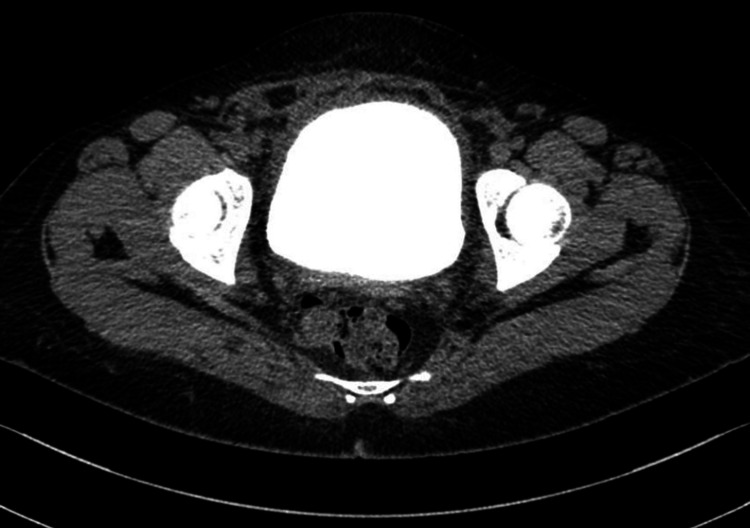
Axial view of the repeated CT cystogram after four weeks from the initial study showing a fully opacified, well-rounded urinary bladder with no evidence of contrast leakage and resolution of the anterior pelvic collection.
